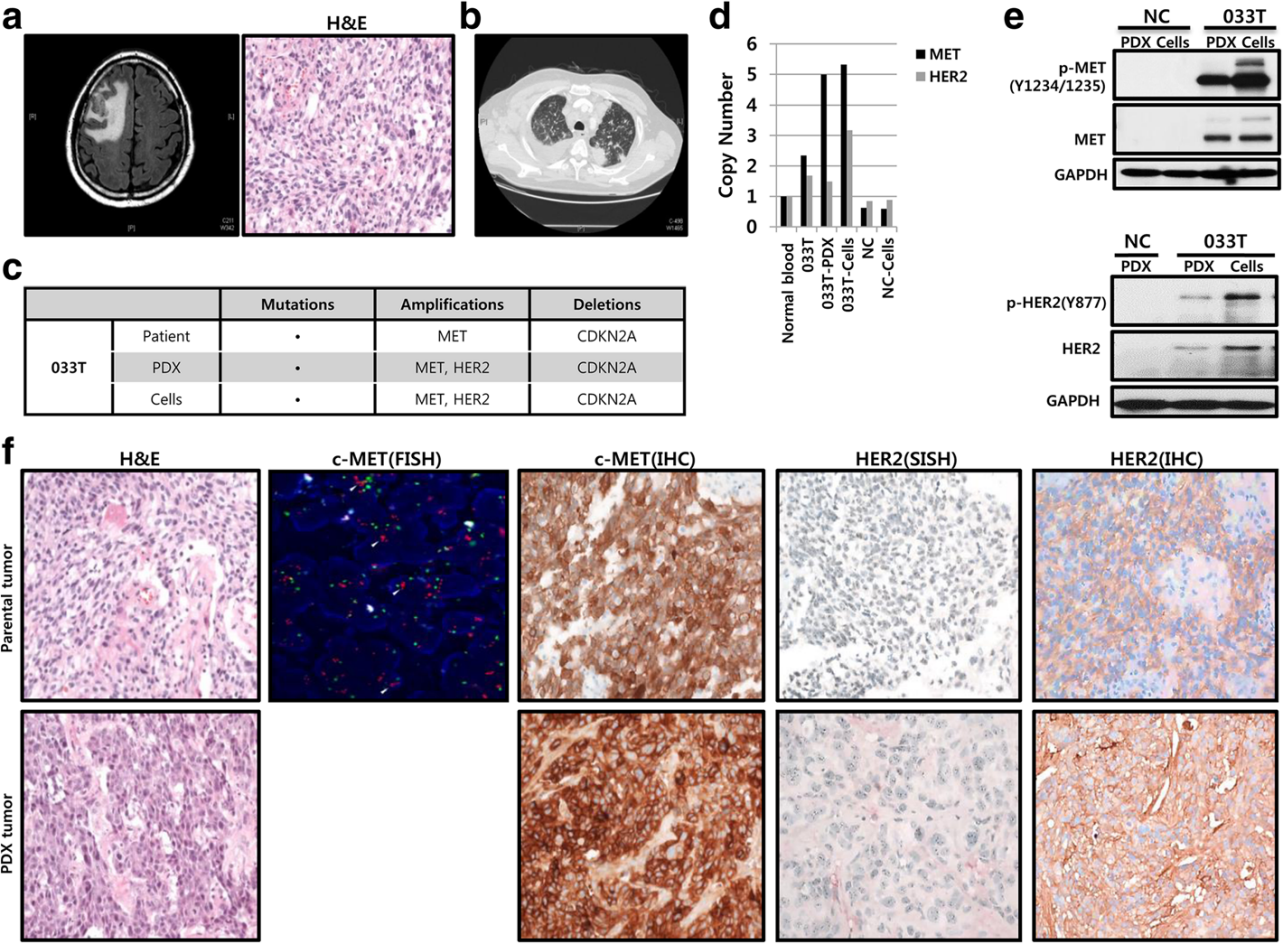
MET and HER2 expression levels in 033 T tumor sample. a Brain MRI showed a relatively well-demarcated enhancing mass in right frontal lobe. b Chest CT showed multiple masses, measuring up to 4 cm, in 033 T patient. c Genomic profiling of focal amplifications in 033 T patient derived tumor, PDXs, and PDX cells identified focal gains of *MET* and *HER2.*
